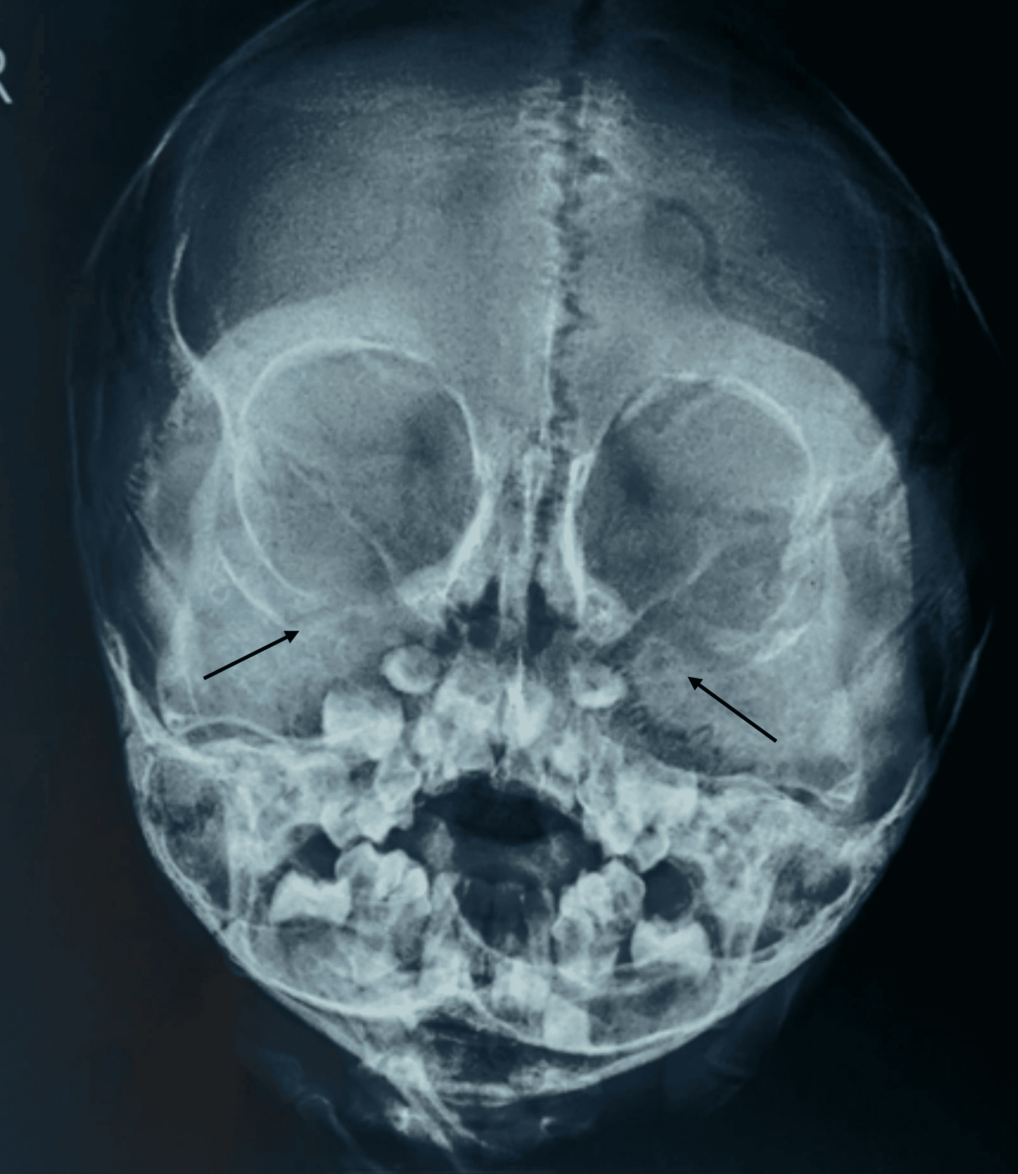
X-ray skull reveals homogenous enhancing masses in bilateral maxillary sinuses with extension into orbits (indicated by arrows)
